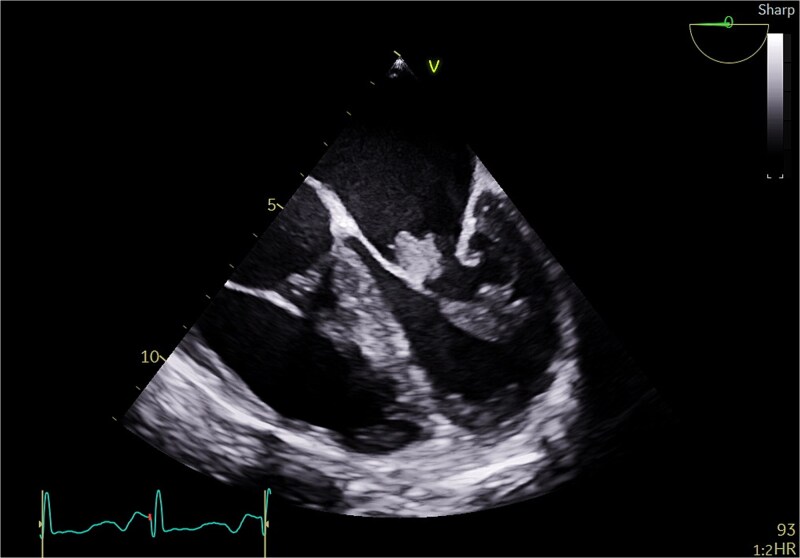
Transesophageal echo appearance of mass attached to posterior leaflet of mitral valve (mid esophageal 4 chamber view).
